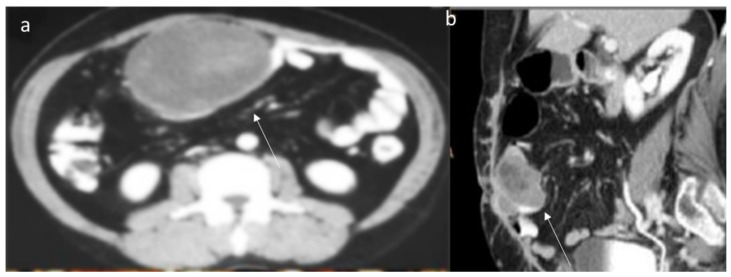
Ileal Gist (arrow) in CT portal phase of contrast study ((a) axial plane; (b) MPR sagittal plane). The lesion shows moderate enhancement with central necrosis.
